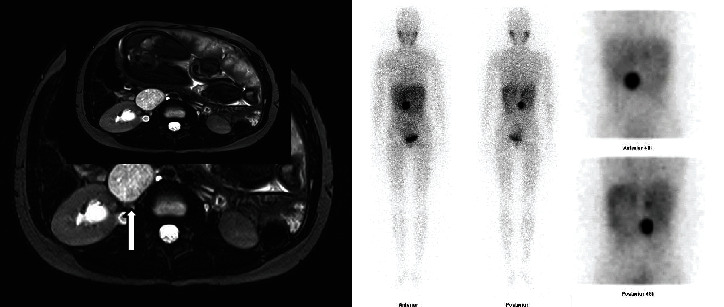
Case 1. (a) Abdominal MRI showing a 41 × 33 × 42 mm retroperitoneal mass with T2 enhancement near the right kidney. (b) Postpartum [^131^I]-metaiodobenzylguanidine (MIBG), showing basal and post-48-hour uptake (anterior and posterior) compatible with a right kidney paramedian paraganglioma.
